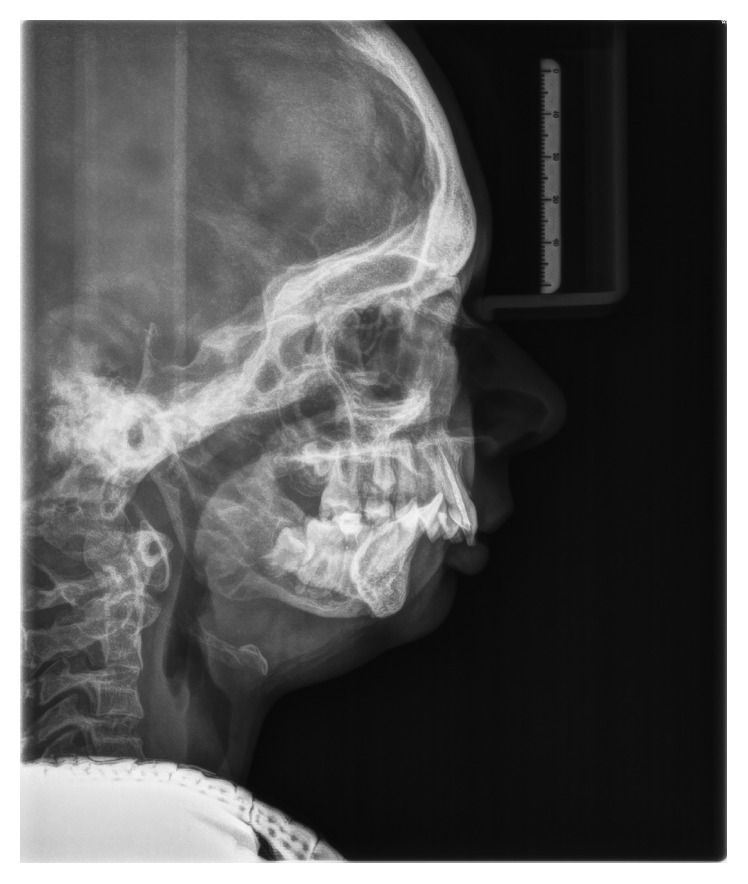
Cephalometric radiograph.
